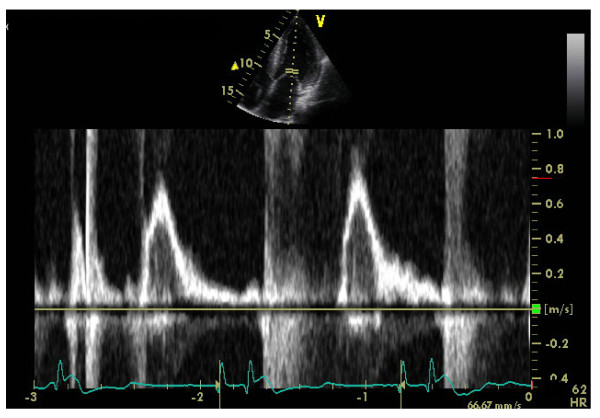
Doppler: near normal systolic function (same patient as in figure 10). Courtesy: Pr Albert A. HAGEGE, University René Descartes, Paris, France.
